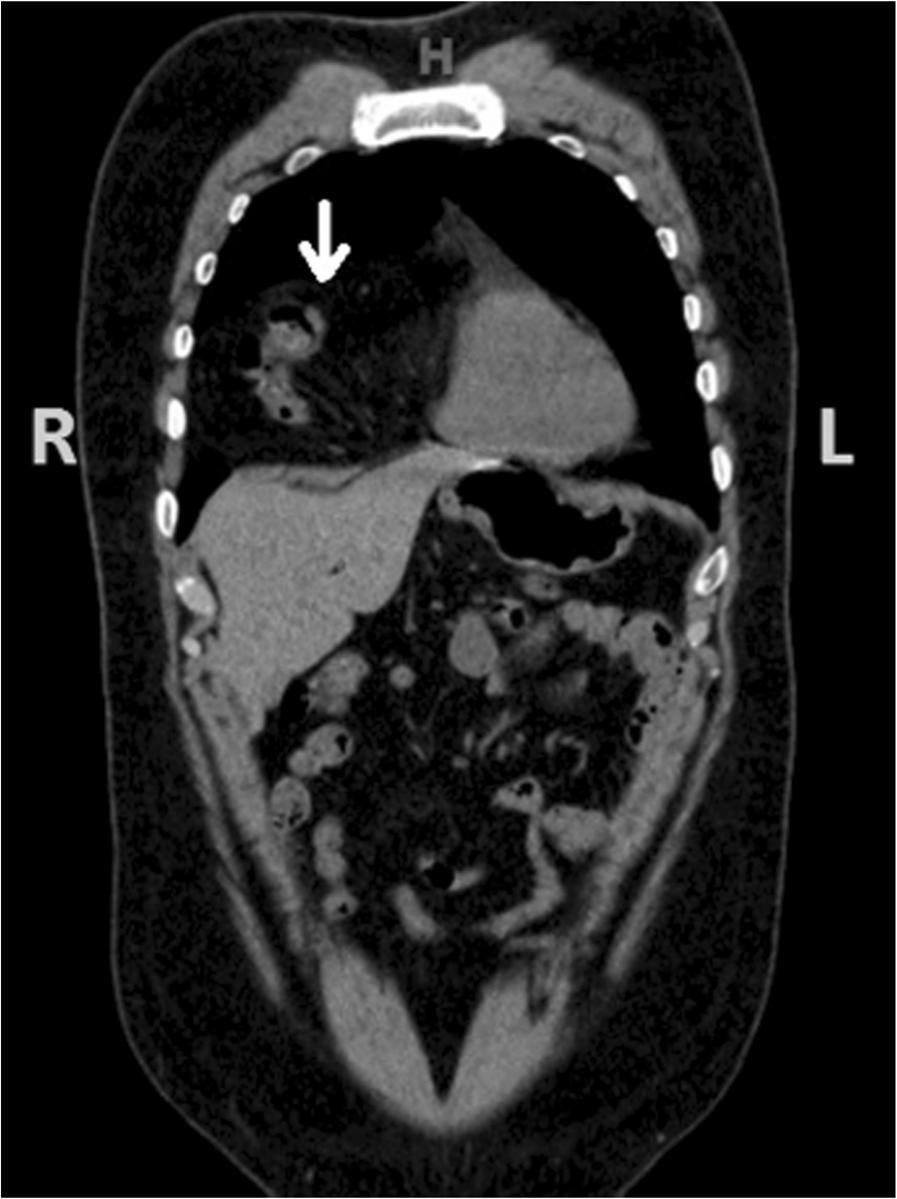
Computed tomographic scan (coronal section) with *arrow* showing bowel loops herniating through the right side of the diaphragm
